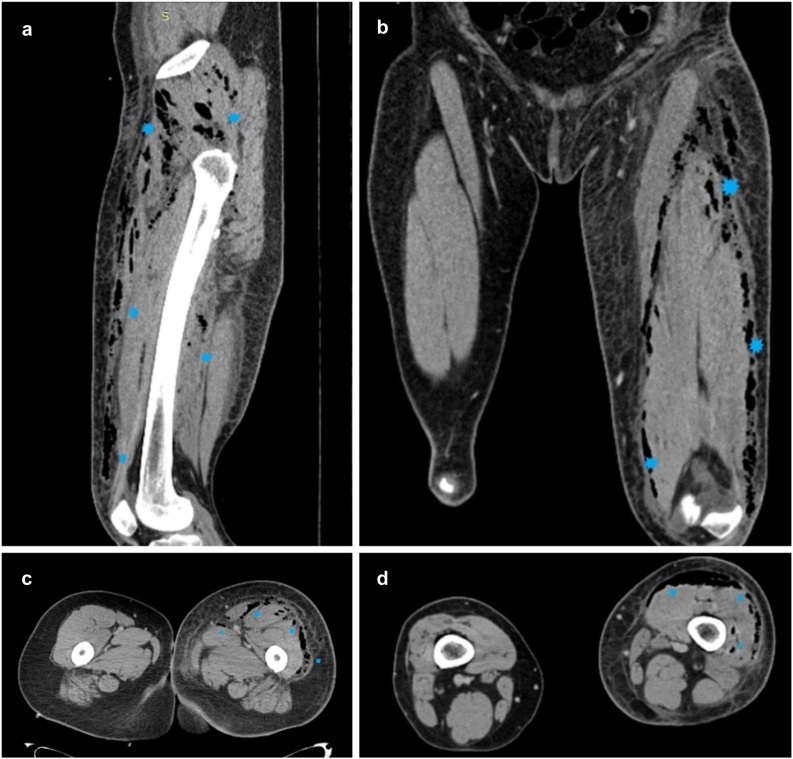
Sagittal (a), coronal (b) and axial (c, d) CT images of the left thigh. *Blue stars* mark multiple gas density areas (phlegmon) distributed mainly in soft tissues of anterior thigh compartment and gluteal region.
